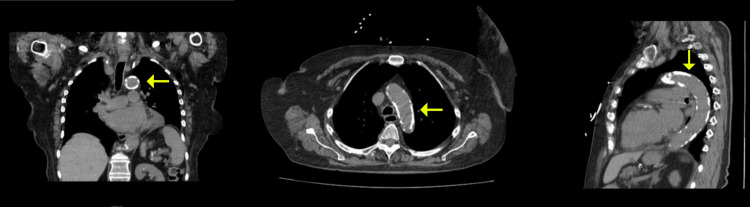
Chest Computed Tomography (CT) Scan Chest CT scan revealed diffuse calcification of the thoracic aorta (arrows).
